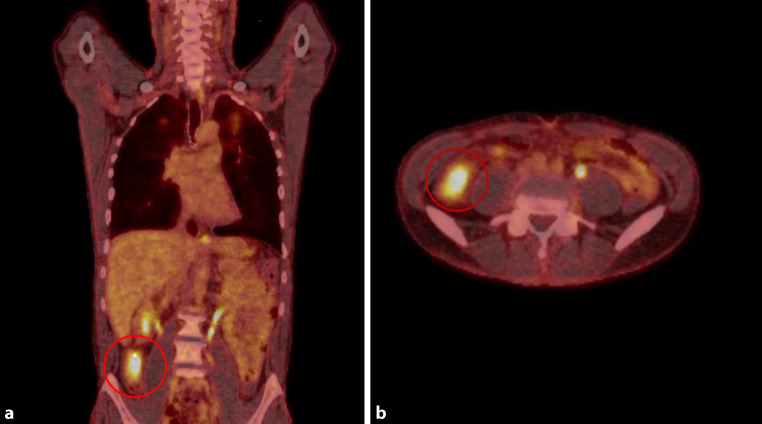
PET CT scan images of the coronal (a) and axial (b) planes showing high metabolic activity in the tumor mass of the cecum (*red circles*) with a maximum standardized uptake value (SUVmax) of 7.9. The high metabolic activity cranial of the tumor in coronal view and at the opposite side of the axial plane is a normal finding and represents parts of the kidney or urinary tract
